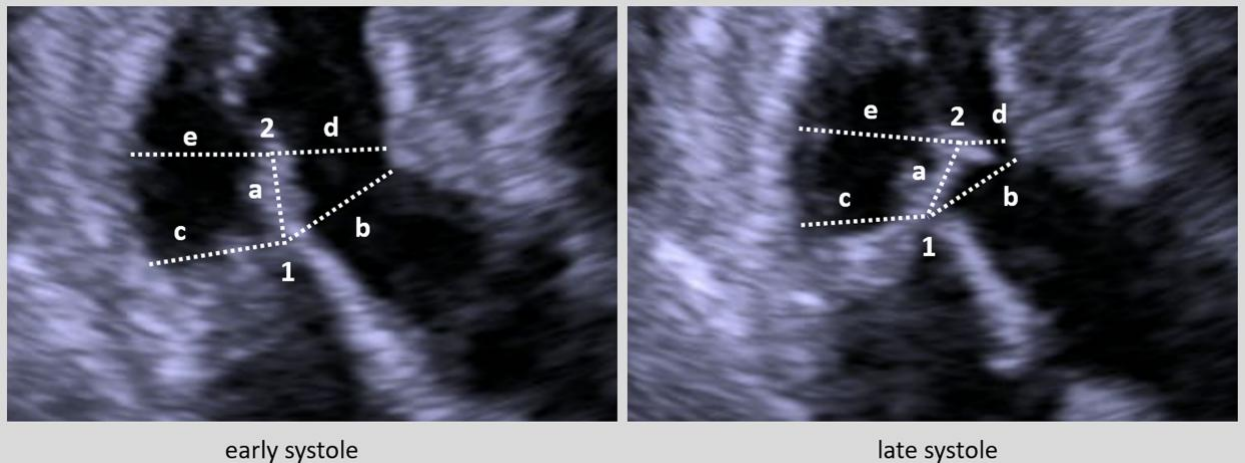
Echocardiographic parameters reflecting the spatial relationship between mitral valve and ventricular walls. (1) Mitral valve coaptation point; (2) mitral leaflet tip; (a) residual leaflet length; (b) closest distance between coaptation point and ventricular septum; (c) closest distance between coaptation point and posterior wall; (d) closest distance between mitral leaflet tip and ventricular septum (TIS); (e) closest distance between mitral leaflet tip and posterior wall.
